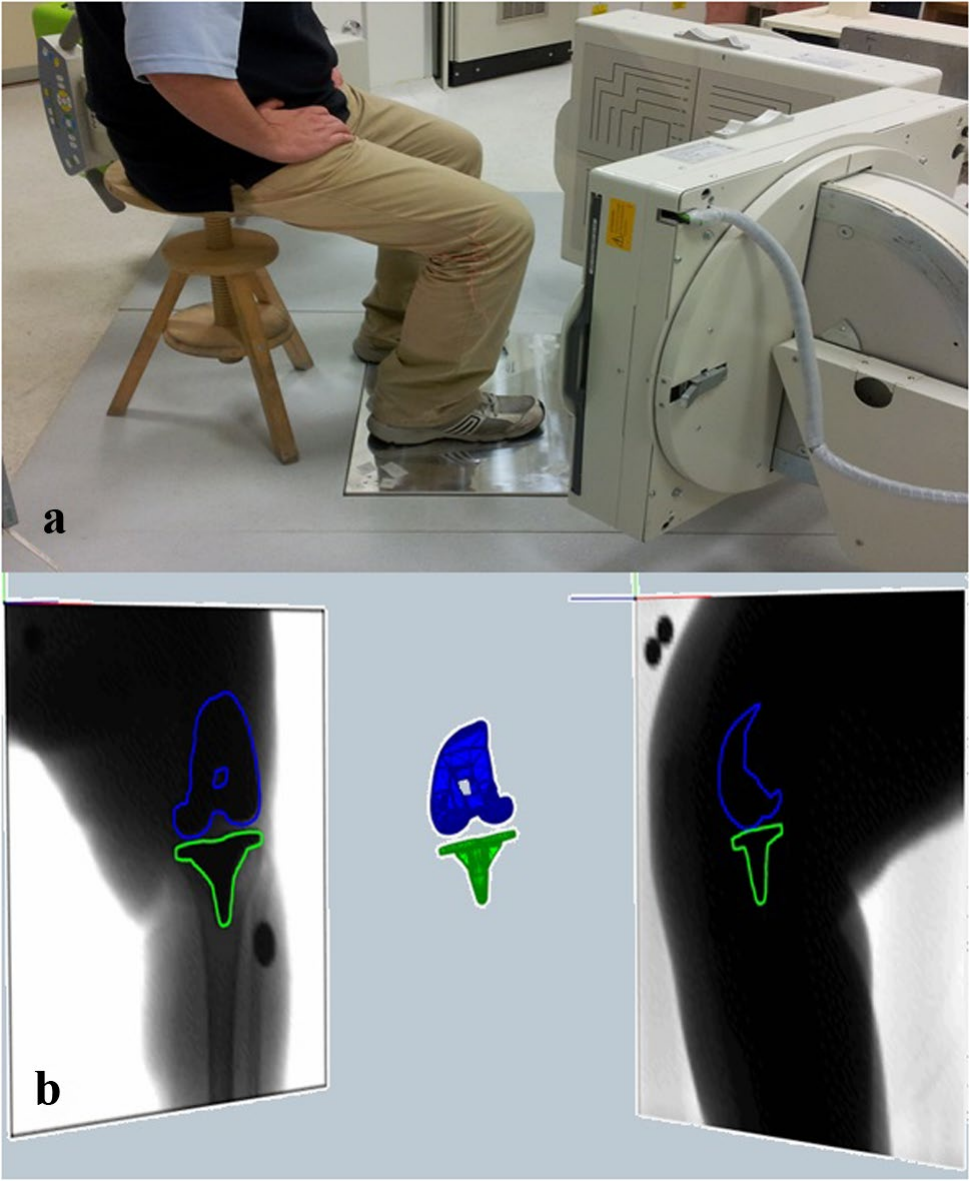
Setup of dynamic radiostereometric analysis (RSA). a RSA setup for the sit-to-stand motor task. b 3D environment of the RSA software for data processing, with contours segmentation on the radiographic images and semi-automatic model positioning
